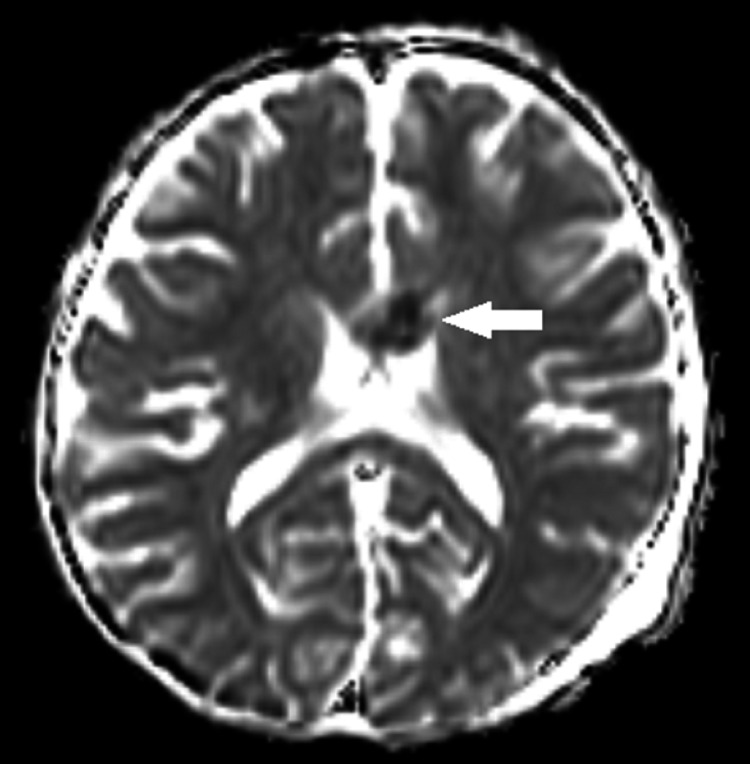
Axial MRI brain ADC image showing hypointense signal (white arrow) involving the genu of corpus callosum (corresponding to high signal on DWI) consistent with infarct ADC: apparent diffusion coefficient; DWI: diffusion-weighted imaging
